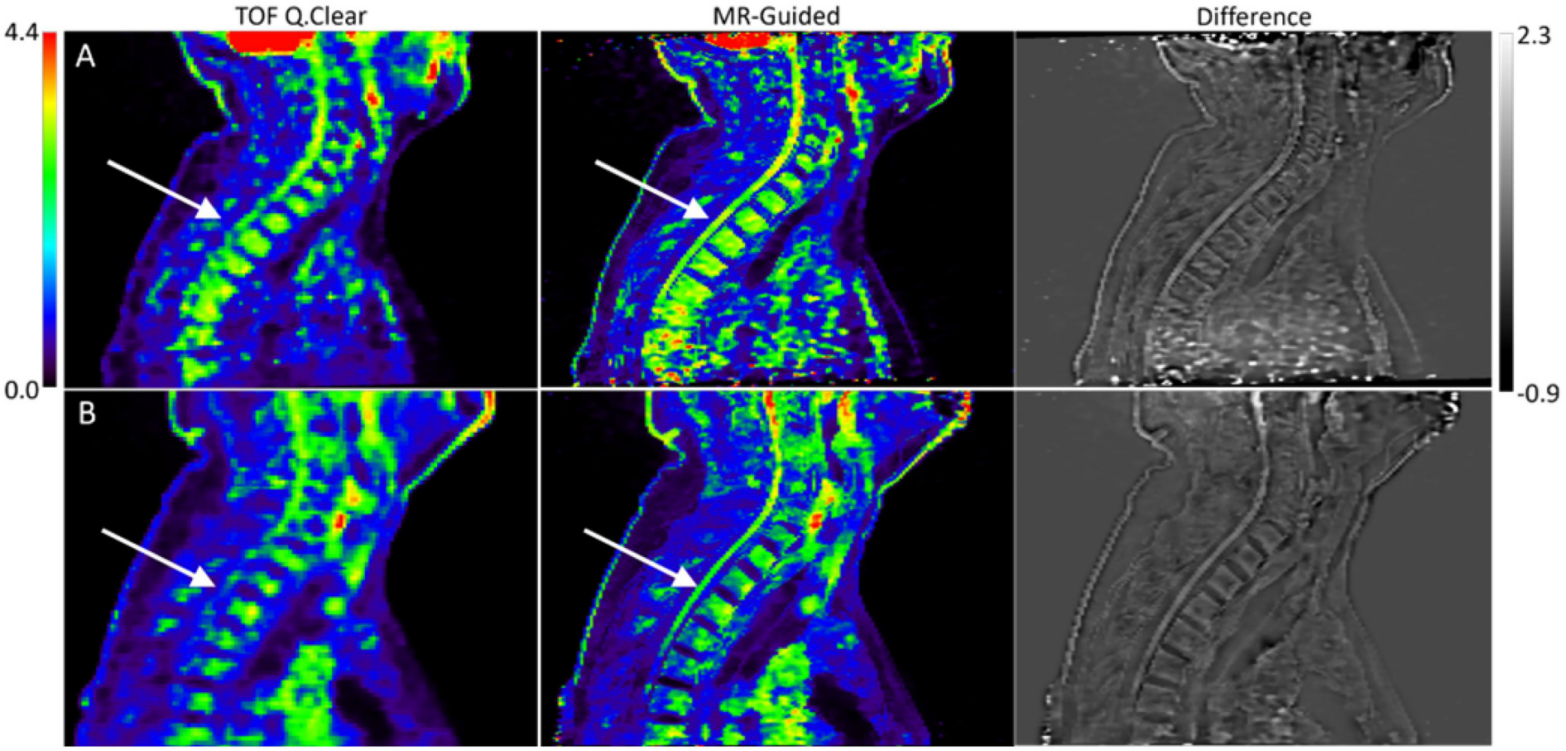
[^18^*F*]FDG PET images of a volunteer [row (A)] and Amytrophic Lateral Sclerosis (ALS) patient [row (B)] reconstructed with TOF Q.Clear, MR-guided TOF Q.Clear and showing a difference image betweens the reconstruction algorithms. The white arrow indicates the spinal cord in the thoracic spine, more clearly visualised in the MR-guided Q.Clear reconstructed images compared to TOF Q.Clear images.
